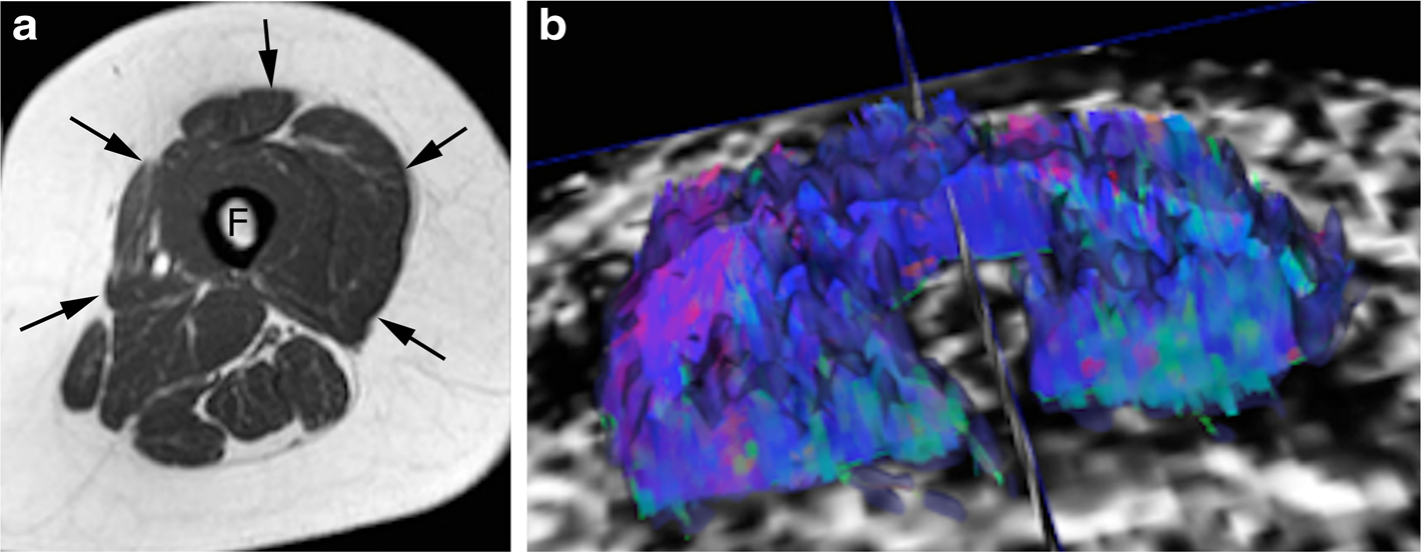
Magnetic resonance of the thigh performed on a 42-year-old male aptient with mild limb-girdle muscular dystrophy. a Morphological appearance on axial T1-weighted image (*arrows*). *F* femur. b The corresponding tractography image shows fibres which are decreased in number, length and organisation due to partial fatty replacement. This appearance can be particularly appreciated if compared to Fig. 1b, which shows a normal participant
